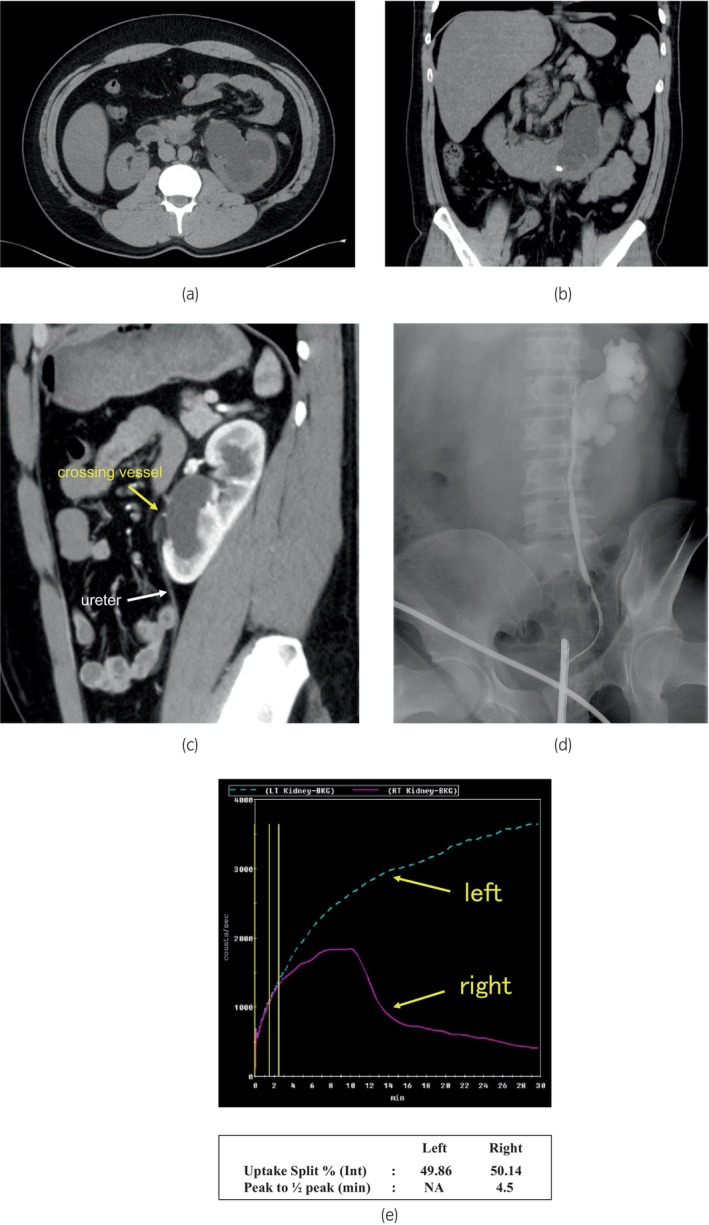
Imaging findings. *Preoperative computed tomography*: The left renal pelvis was dilated (a), and the lower poles of the kidneys were fused on the ventral side of the abdominal aorta to form horseshoe kidney (b). In addition, sagittal scans showed crossing vessels that appeared to be the cause of obstruction (c). *Retrograde pyelography*: It was unclear whether the urinary tract was displaced by the kidneys on retrograde pyelography (d). *Technetium‐99m‐mercaptoacetyltriglycine diuretic renography*: Diuretic renography performed using technetium‐99m‐mercaptoacetyltriglycine (99mTc‐MAG3) showing substantially delayed left renal excretion (e).
